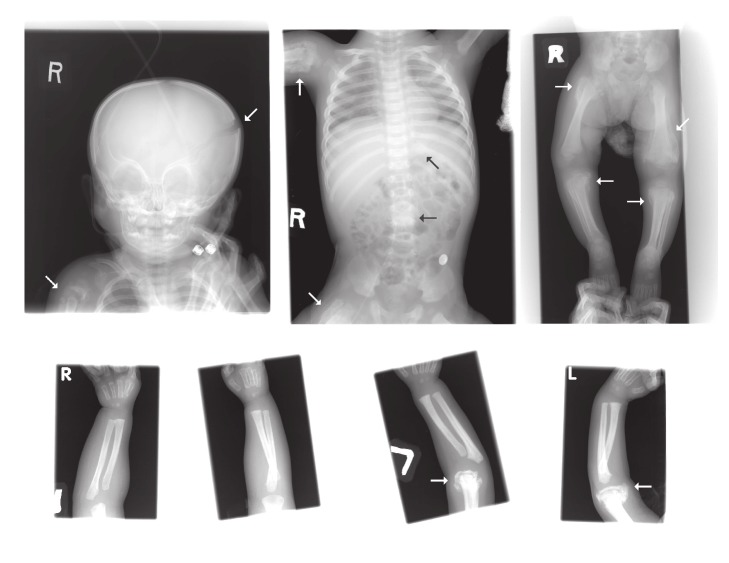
X-ray: a series of plain film radiographs demonstrated calvarial fracture lines at left temporoparietal region, bony anomaly of the spine, multiple old fractures with callus formation involving posterior aspect of left 10, 11th ribs, right proximal humerus, bilateral proximal femurs, and metaphyses of tibias (arrows). Adjacent soft tissue swelling is evident. The above findings were consistent with child abuse.
